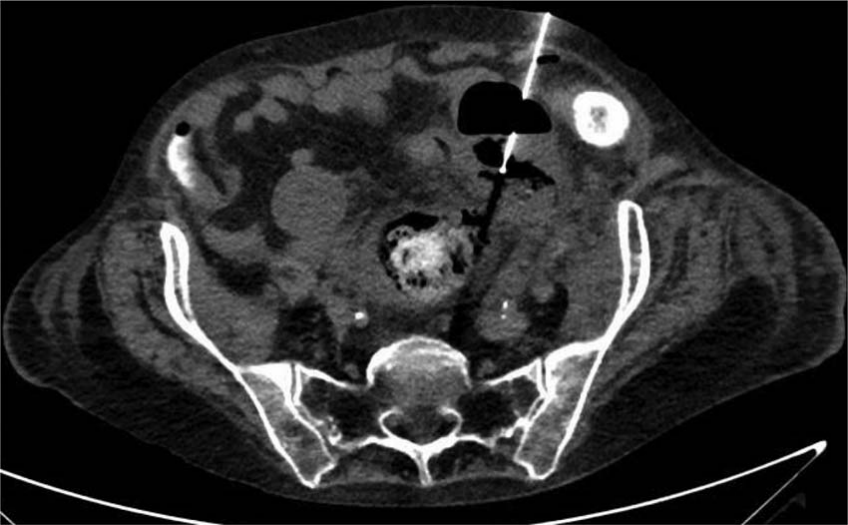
CT-guided percutaneous drainage of abscess.
